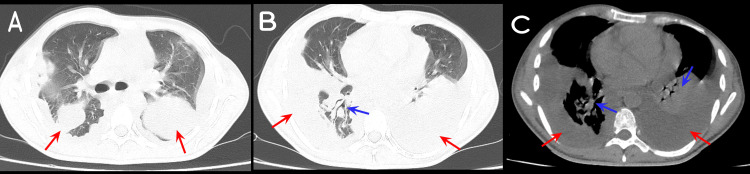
Non-enhanced CT scan of the chest in Case 2 showing (A, B, C) a moderate amount of bilateral loculated pleural effusion (red arrows) with (B, C) bilateral lung consolidation (blue arrows). A, B, lung view; C, mediastinal view
